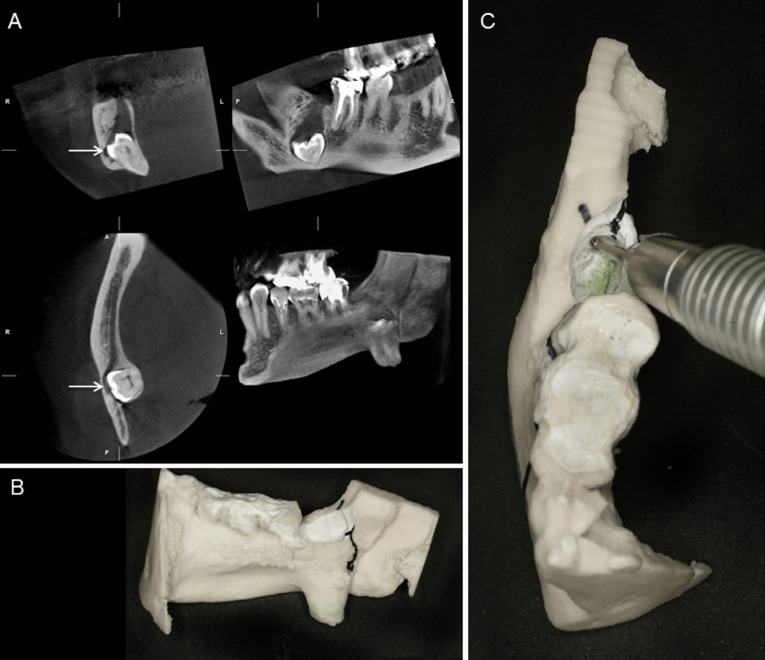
A) Follicular cyst of the right lower third molar has caused severe dislocation of the tooth. In the CBCT images the mandibular canal is marked with an arrow. B) and C) With the help of the model it was possible to plan the operation and it was used for surgical training.
